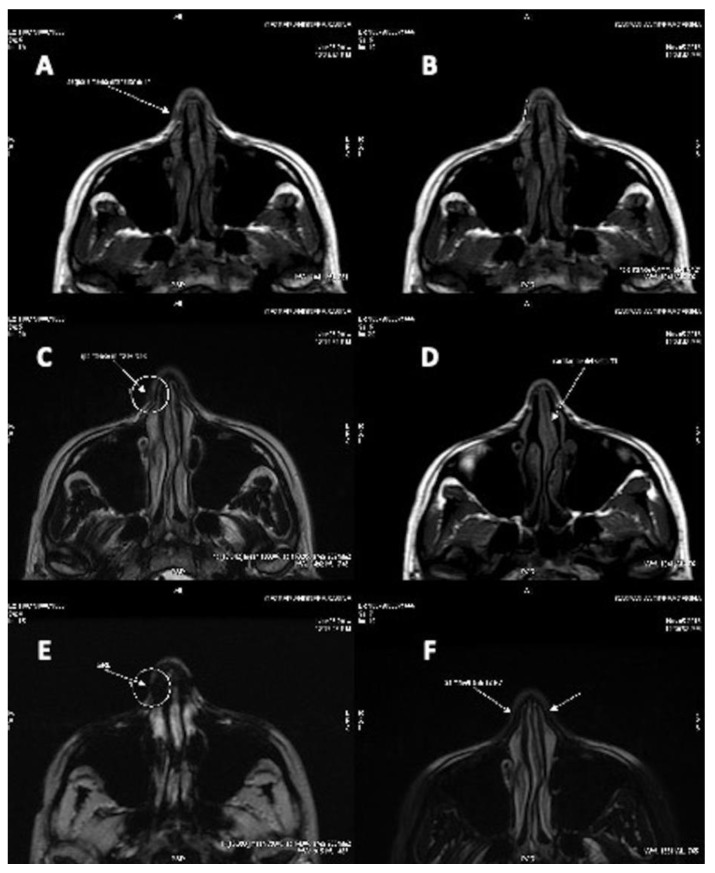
Computerised tomographic (CT) scans of patient showed in Figure 4. (A,C,E) Pre-operative situation. (A) CT scans, T1 medium signal intensity, showed the pre-operative situation with soft tissue defect of the nasal tip and nasal septum deviation. (B) CT scans, T1 medium signal intensity, showed the post-operative situation with an improvement of soft tissue volume in the treated area. (C) CT scans, T2 high signal intensity, showed the pre-operative situation in detail, with cartilage defect and nasal valve collapse. (D) CT scans, T2 high signal intensity, showed the post-operative situation with correction of the nasal valve collapse. (E) CT scans, T2 high signal intensity, showed the detail of the pre-operative situation with soft tissue defect of the nasal tip and cartilage defect in detail. (F) CT scans, T2 high signal intensity, of the same area after 12 months show the regenerated site in the post-operative image with soft tissue volume improvement and the correction of the nasal septum deviation.
